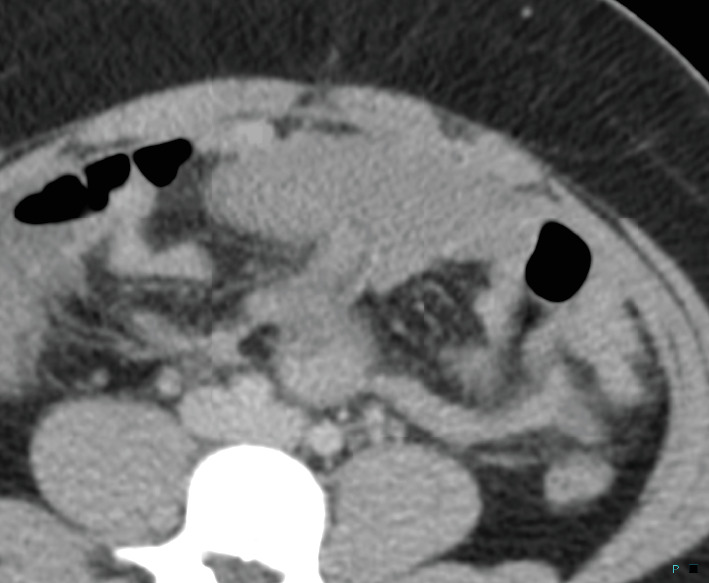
Enlarged image of CT scan demonstrates torsed pedicle of the spleen located in the anterior abdomen.
